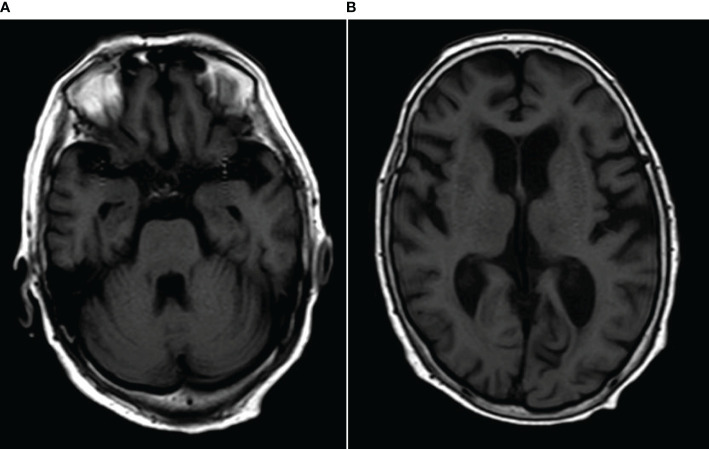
(A, B) T1-weighted images revealed low signal intensity in the pons and bilateral thalamus.
